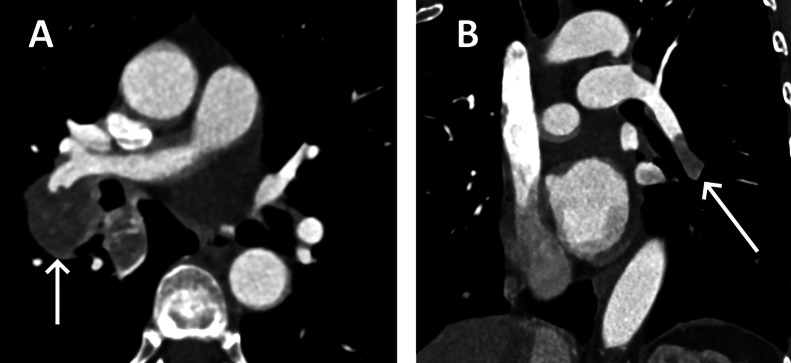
Contrast-enhanced CT of the lungs showing enlarged hilar lymph nodes (A, arrow) and embolism in a segmental pulmonary artery that was later shown to be tumoral in nature (B, arrow).
